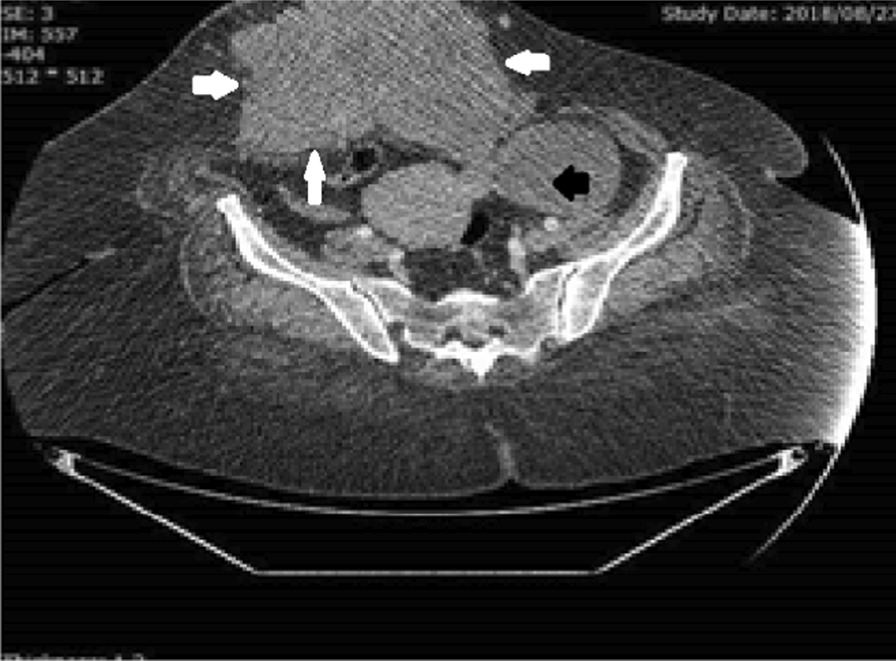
Preoperative pelvic computed tomography scan of patient 2. Borders of the abdominal wall mass are marked by white arrows. The black arrow indicates the pelvic mass
